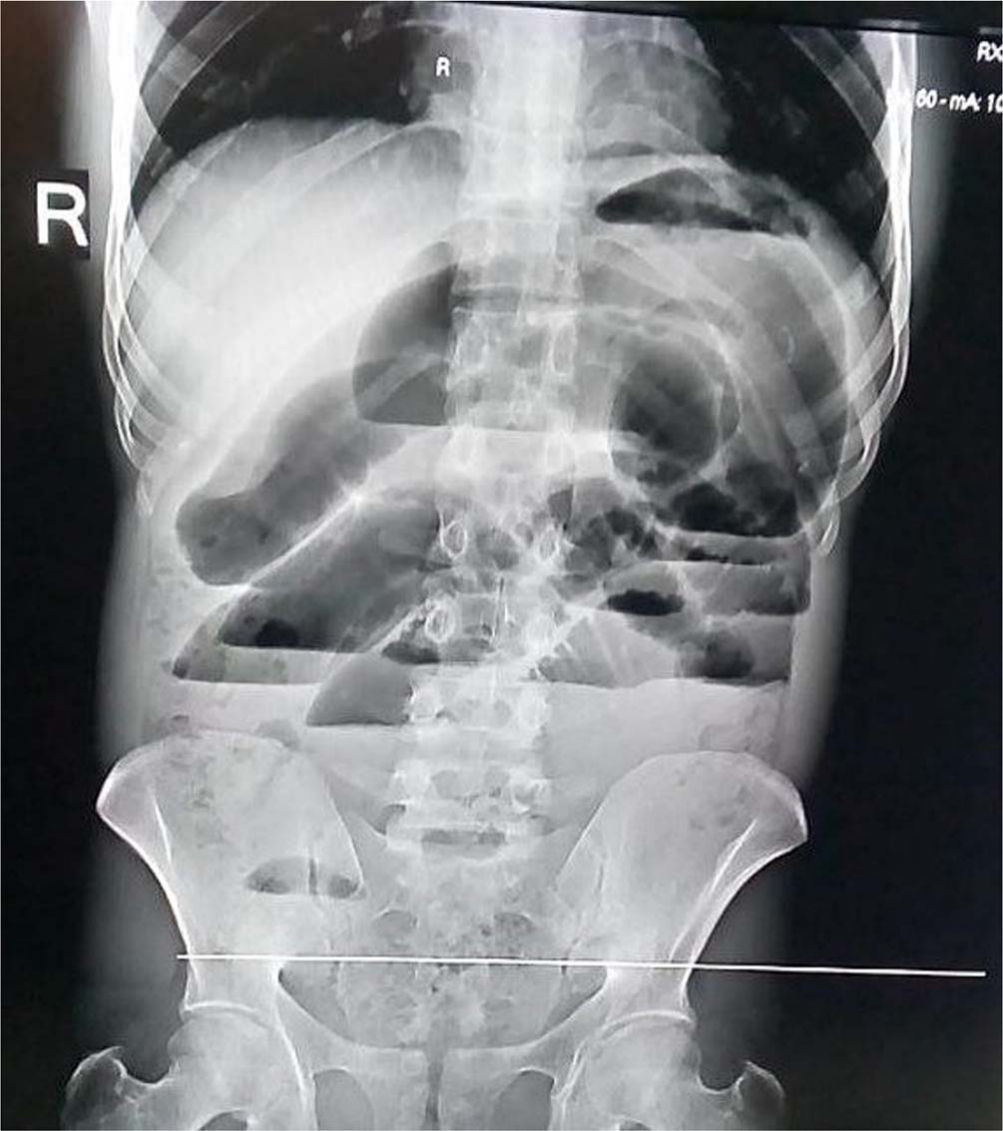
Abdominal X-ray—multiple air fluid levels.
